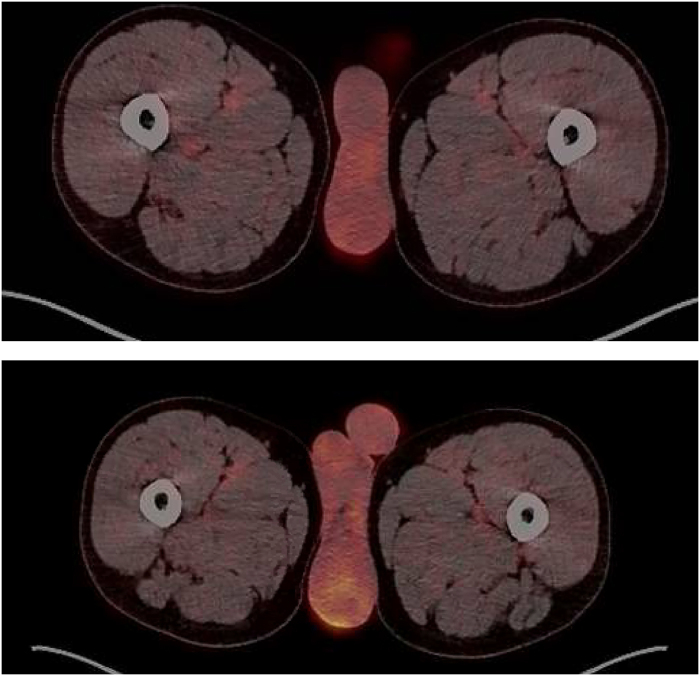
Positron emission tomography–computed tomography image of the scrotum: (a) normal very low-level physiological uptake in the testicles bilaterally; (b) increased uptake in the left epididymal tail within the leiomyoma.
